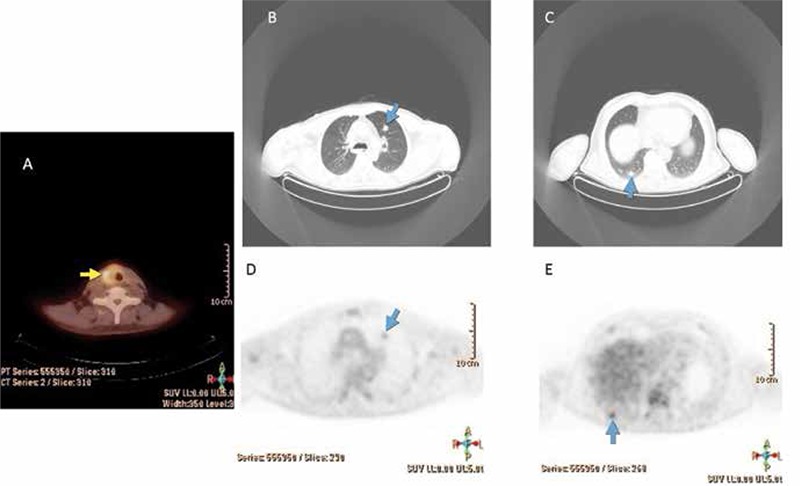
F-18 FDG PET/CT images of a high risk patient with papillary thyroid cancer with folicular variant. Increased FDG uptake was seen in a right thyroid lobe mass invading adjacent soft tissue structures (A, yellow arrow). There are also left and right small lung nodules which show increased FDG uptake (B, C, D, E, blue arrows)
